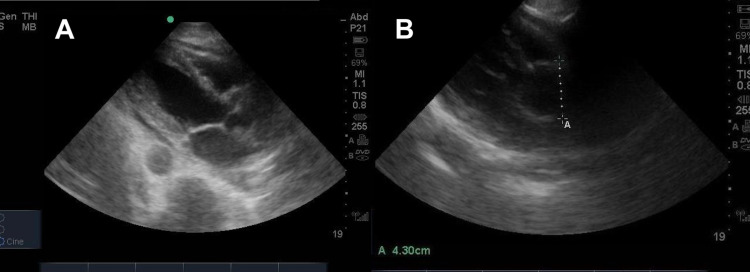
2A Bedside echocardiogram demonstrating pericardial effusion without evidence of tamponade 2B Bedside echocardiogram demonstrating widening of the aortic root measuring 4.3 cm in diameter
